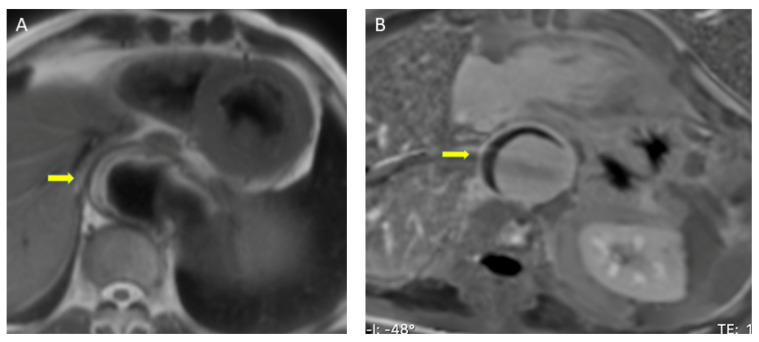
Magnetic resonance imaging assessment of intramural hematoma (arrows). (A) Spin-Echo sequence. The eccentric thickening of the aortic wall has a high T1 signal, eliminating the possibility that it is an acute stage. (B) Late gadolinium- enhancement sequence. Aortic eccentric wall thickening with no mural enhancement, suggestive of intramural hematoma.
